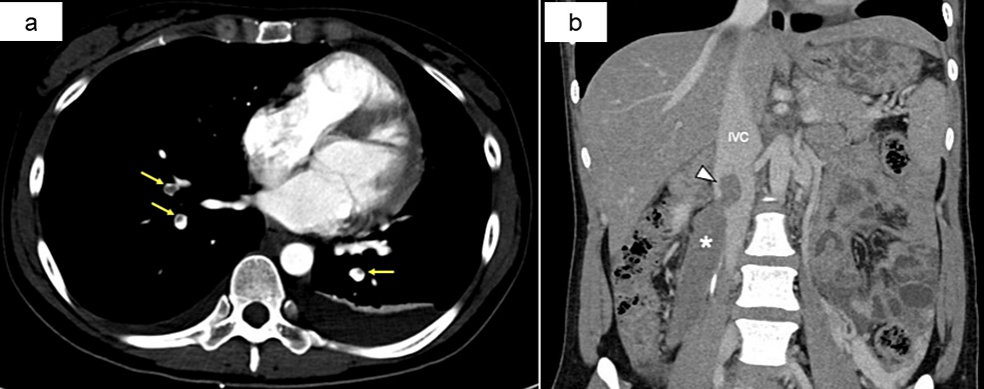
Contrast-enhanced CT just after the cesarean section. (a) Yellow arrows show peripheral pulmonary embolism. (b) The asterisk shows enormous right ovarian vein thrombosis, the size of which was 12×2 cm. The white triangle shows pedunculated thrombi protruding into the IVC. IVC, inferior vena cava; OVT, ovarian vein thrombosis; CT, computed tomography
